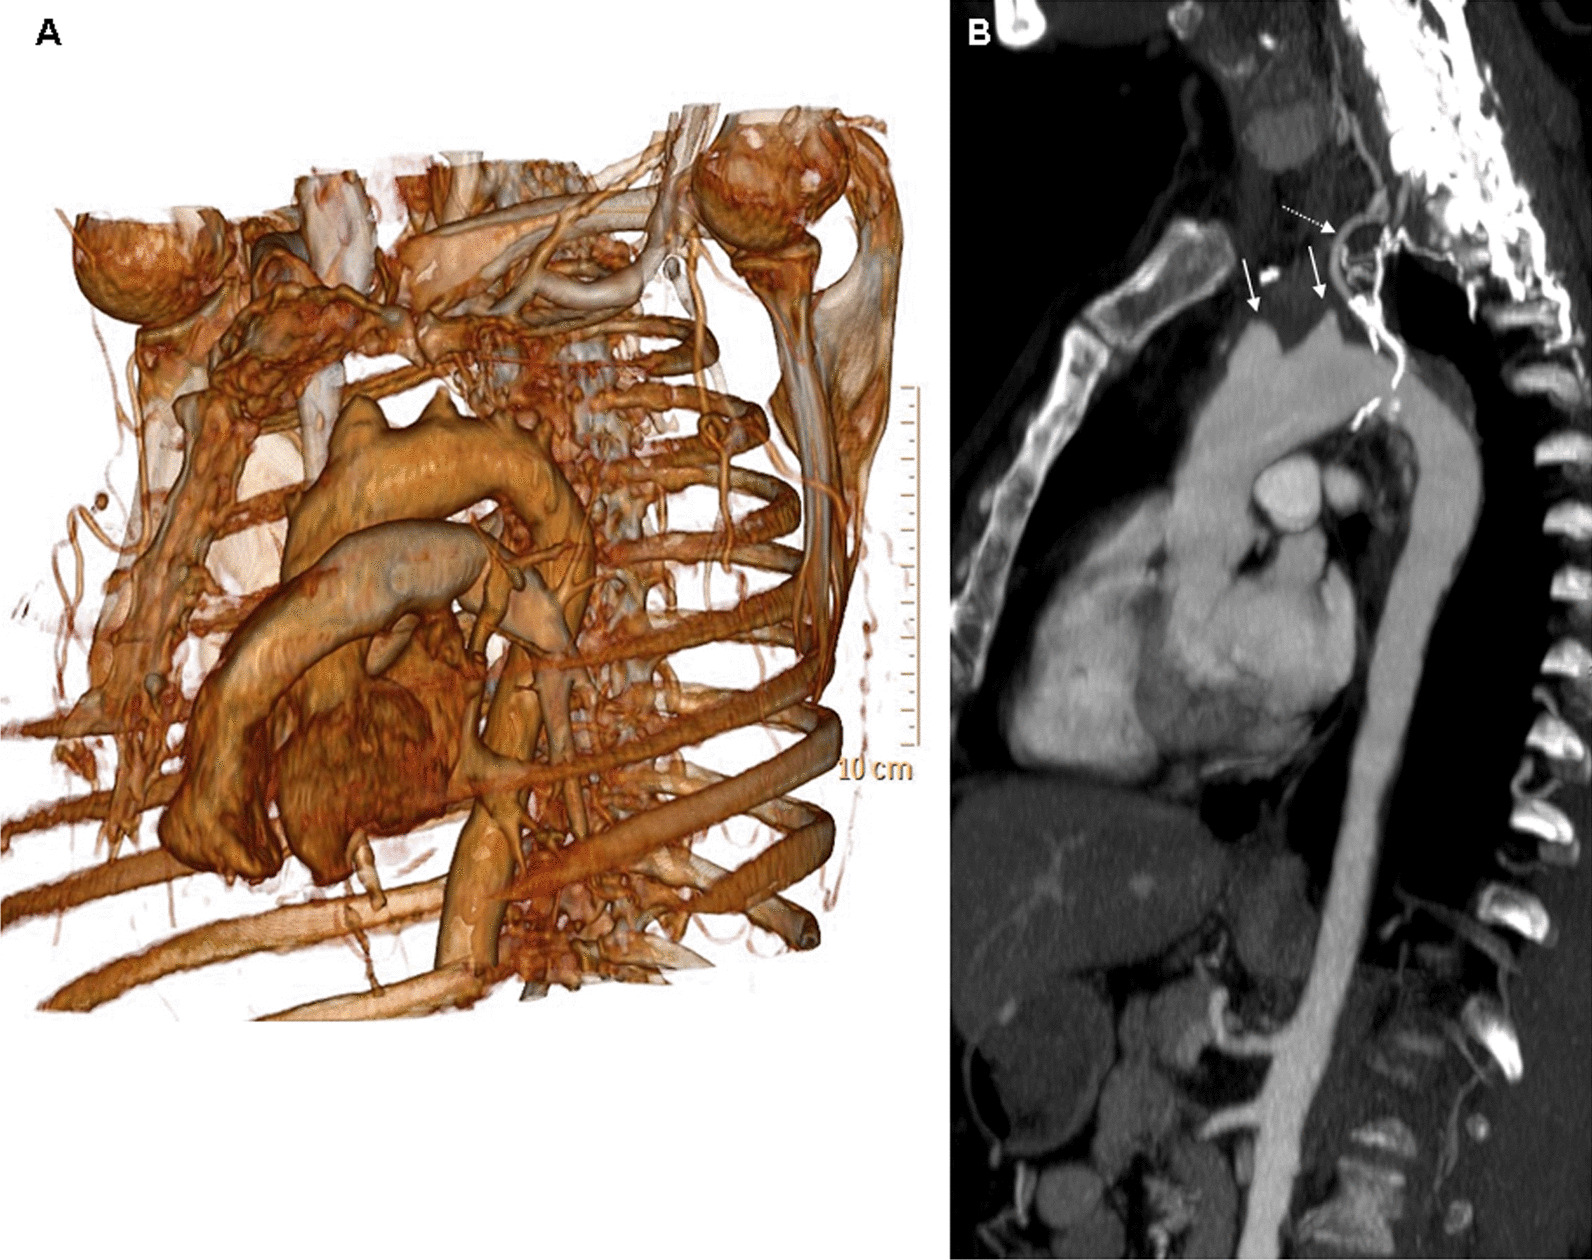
Volume-rendered CTA image, left anterior oblique orientation depicting the supra-aortic vessels occlusion (A). Curved multiplane reformat displaying the occluded supra-aortic vessels (arrows) and the collateral artery (dotted arrow) arising from the right coronary artery feeding the left vertebral artery (B)
